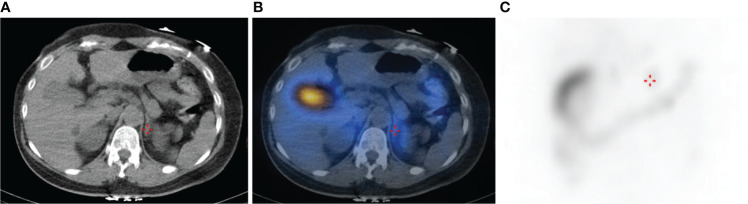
CT and NP-59 SPECT image of the abdomen showing a focus of increased tracer uptake in the region of the left adrenal gland (crosshairs). (A) Abdominal CT image of the axial plane shows a 0.6 cm left adrenal nodule. (B) Fused SPECT/CT image shows focal uptake at the left adrenal gland, indicating aldosterone-producing nodule. (C) Planar image shows mildly intense focal lesion over the left suprarenal area.
